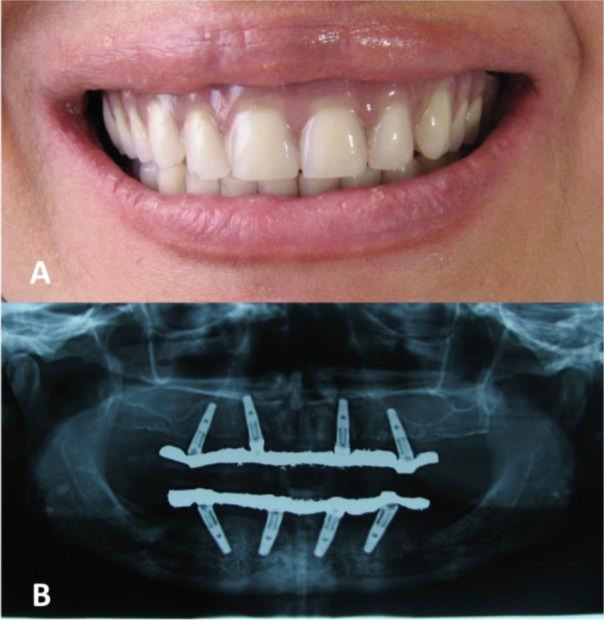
(A) Clinical and (B) radiographic views of metal-resin (hybrid) All-on-4 prostheses
